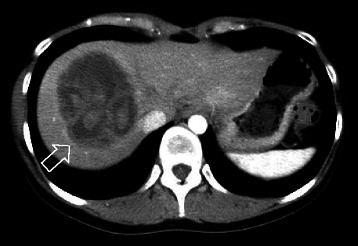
Enhanced computed tomography scan of the abdomen showing the multilocular cystic mass. Enhanced computed tomography scan showing a multilocular cystic mass (arrow) with enhanced septa, measuring 82 × 66 mm in liver segment 8.
